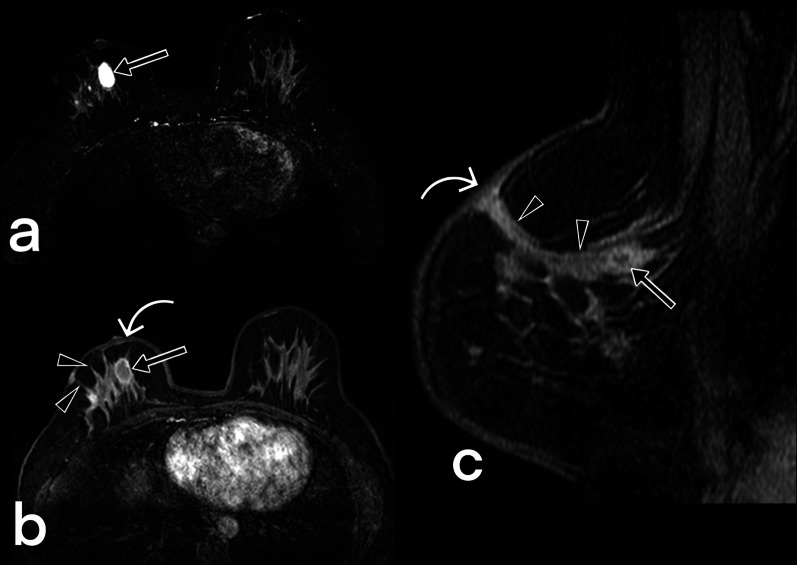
MRI images of a 41-year-old female. Axial T2 weighted fat suppressed (a), fat-suppressed T1 weighted contrast-enhanced axial (b), and sagittal (c) images reveal cystic lesions (arrows) in the right breast with peripheral enhancement, compatible with an abscess that is fistulized (arrowheads) to the thickened skin (curved arrow). BIRADS 4
